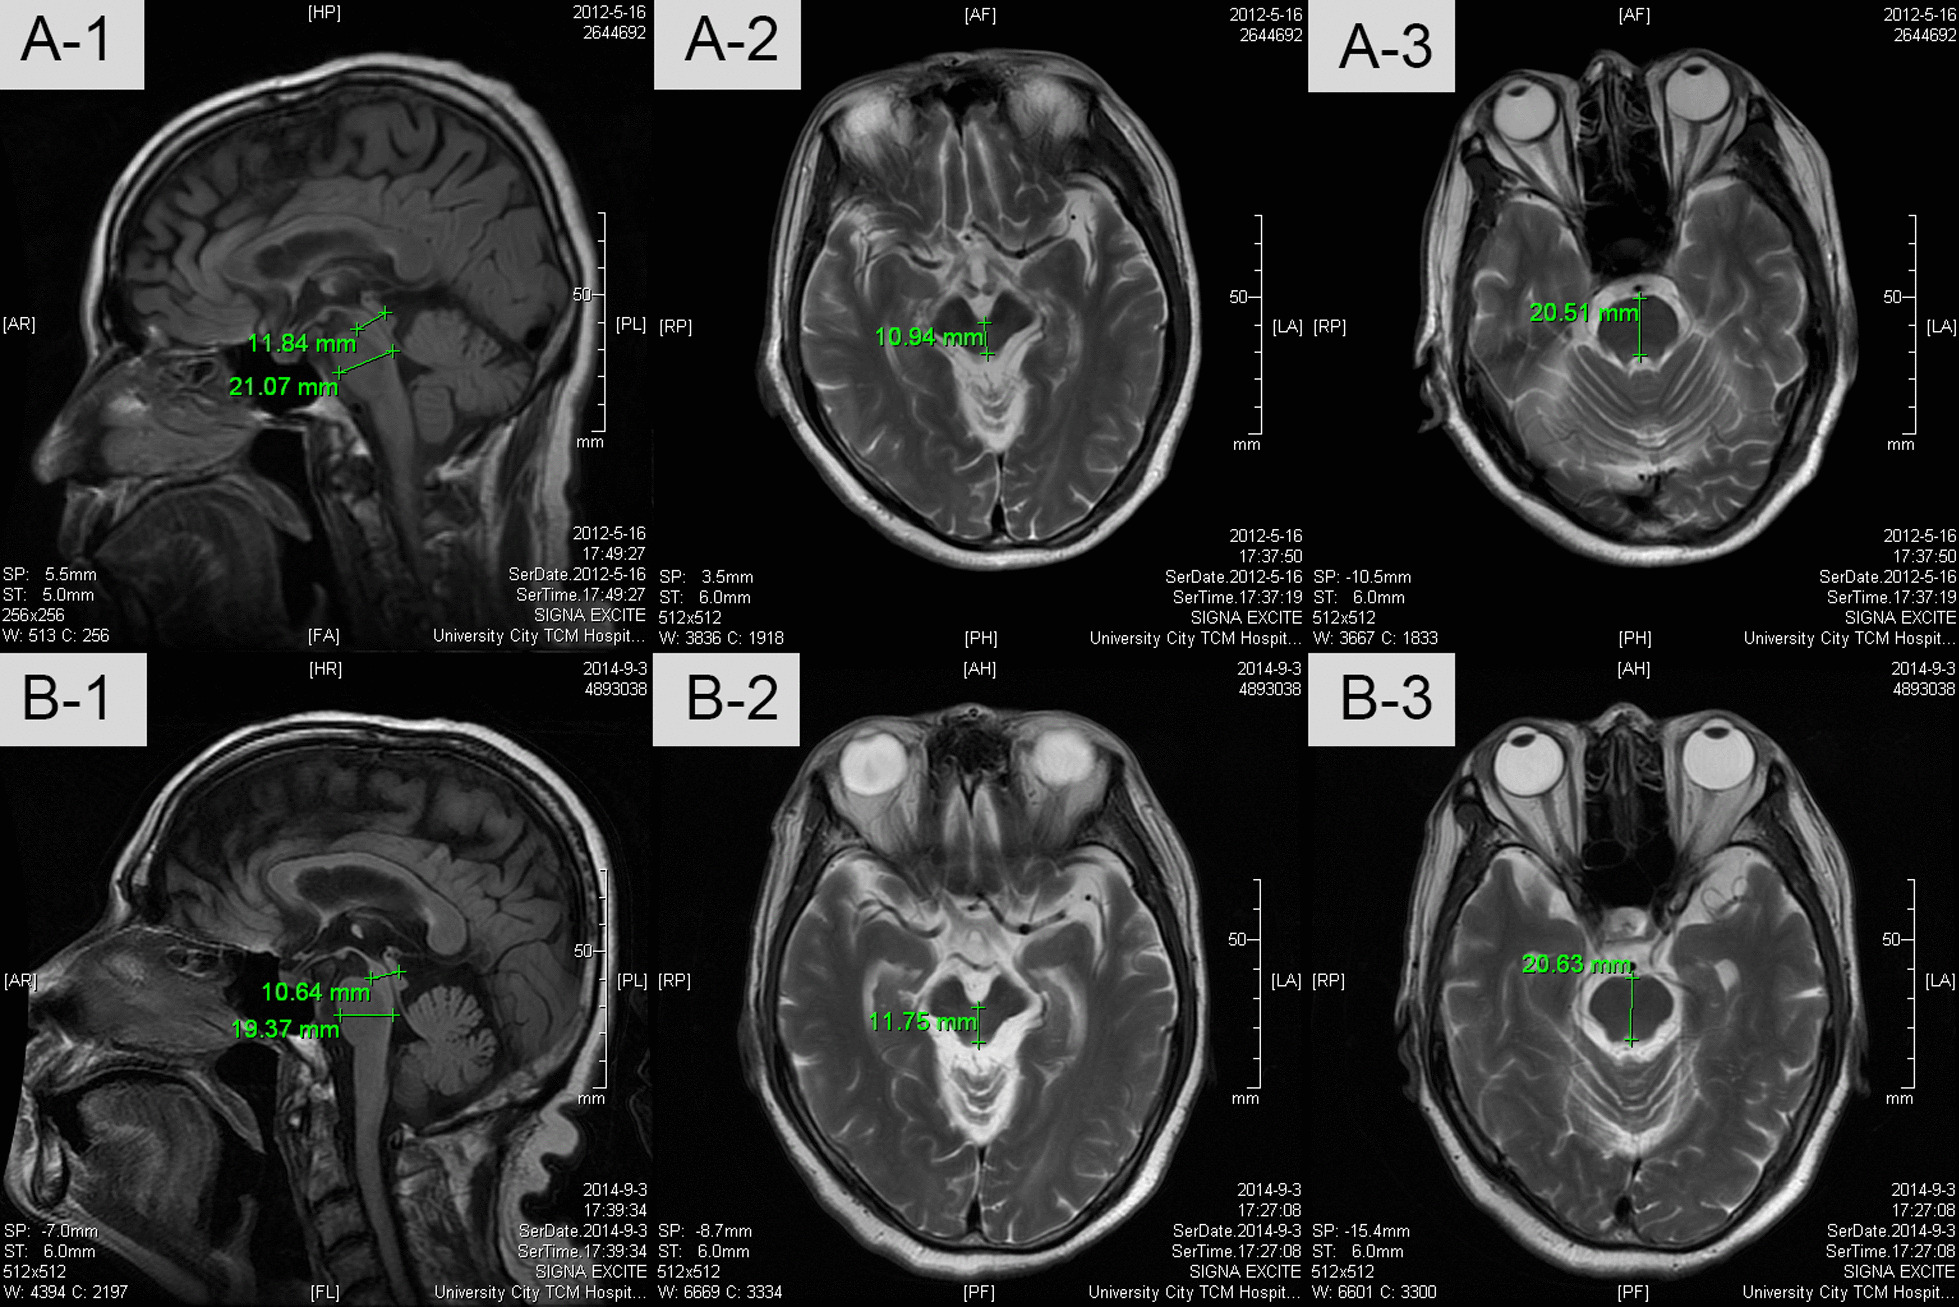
Brain MRI before and after umbilical cord blood stem cells therapy. A 1–3: MRI in May 2012. B 1–3: MRI in September 2014. The atrophy of pons and mesencephalon did not deteriorate 2 years after umbilical cord blood stem cells therapy
